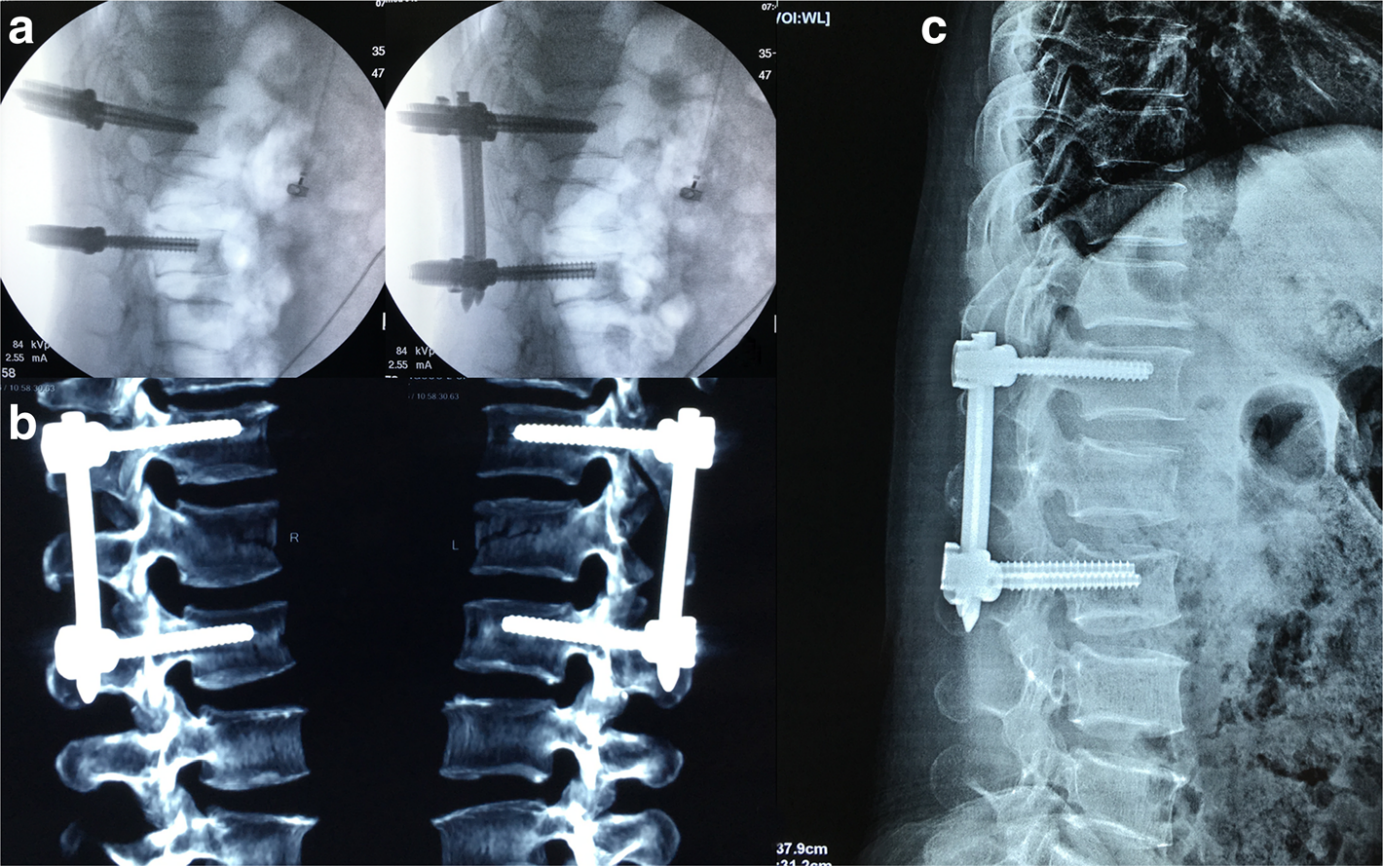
a The intraoperative contrast chart shows the vertebral height restoration and kyphosis correction by using the new screw system. b CT examination 6 months after the operation shows that the internal fixation position was good. c X-ray examination 2 years after surgery shows the reduction in vertebral height, and physiological curvature of the thoracolumbar spine was maintained well. His follow-up radiographic parameters were as follows: VBI, 0.95; HAMFV, 0.97; VBA, 2°; and BCA, 5°
